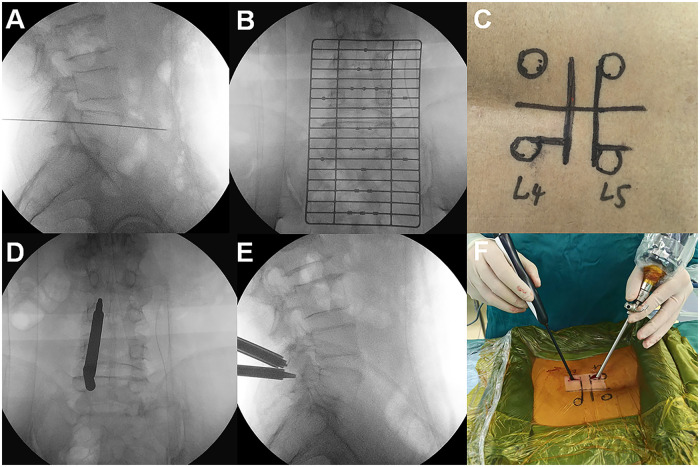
Preparation and safety measures for UBED. (A) To ensure that the surgical level is perpendicular to the ground, a Kirschner needle is employed in a lumbar lateral x-ray. (B) Using a grid-shaped metal marker to mark the anterior and posterior x-ray film; (C) Marking the surface projection of the surgical level, pedicles, and midline of the spinous process; (D,E) Reconfirming the surgical level using fluoroscopy following skin incision and puncture; (F) Using both hands to simultaneously operate the electrical coagulator and endoscope during surgery.
